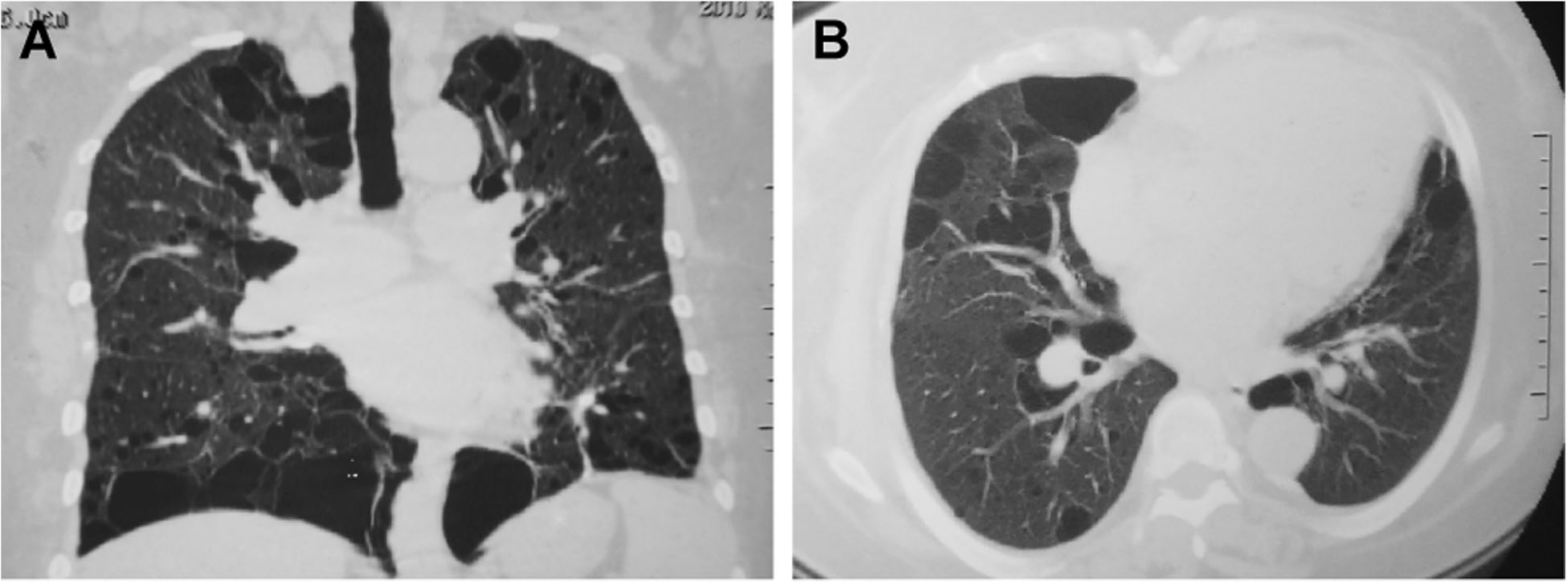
Thoracic CT findings of patients with BHD. a Coronal reformatted image of high-resolution chest CT images showing lower lung predominant, para-mediastinal, and fusiform cysts in BHDs; b Axial high-resolution chest CT images through the lower lungs
